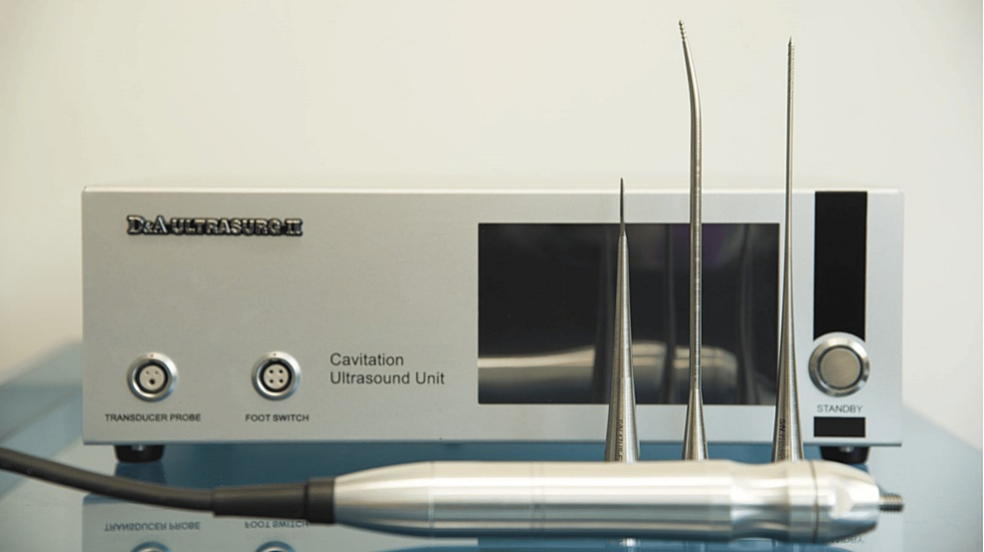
D & A Ultrasurg ultrasound device including the central unit, footswitch, transducer, and probes
